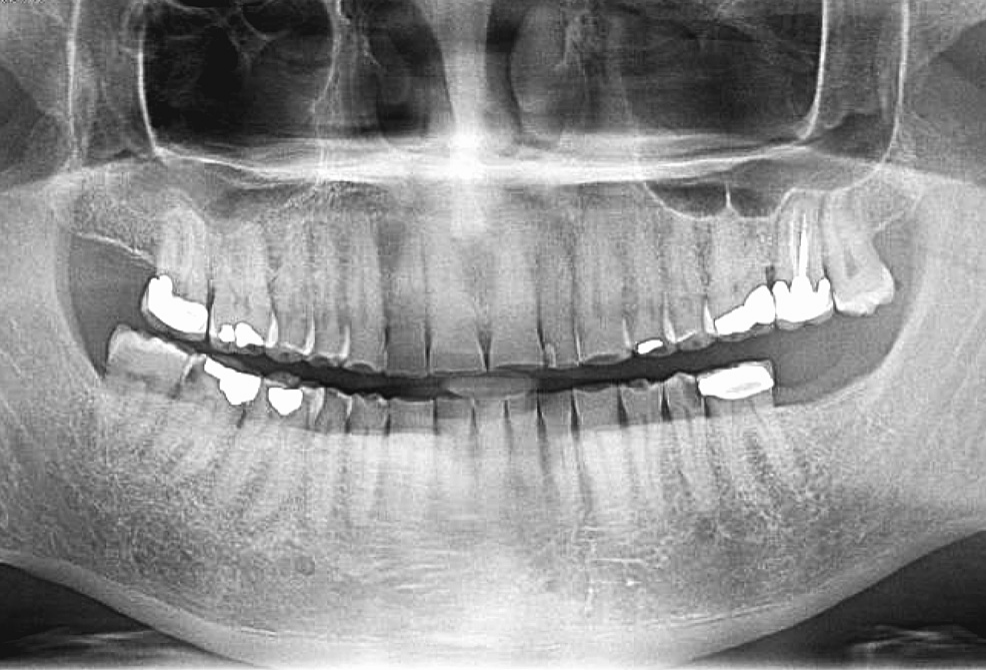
Panoramic x-ray showing no evidence of dental infection.
